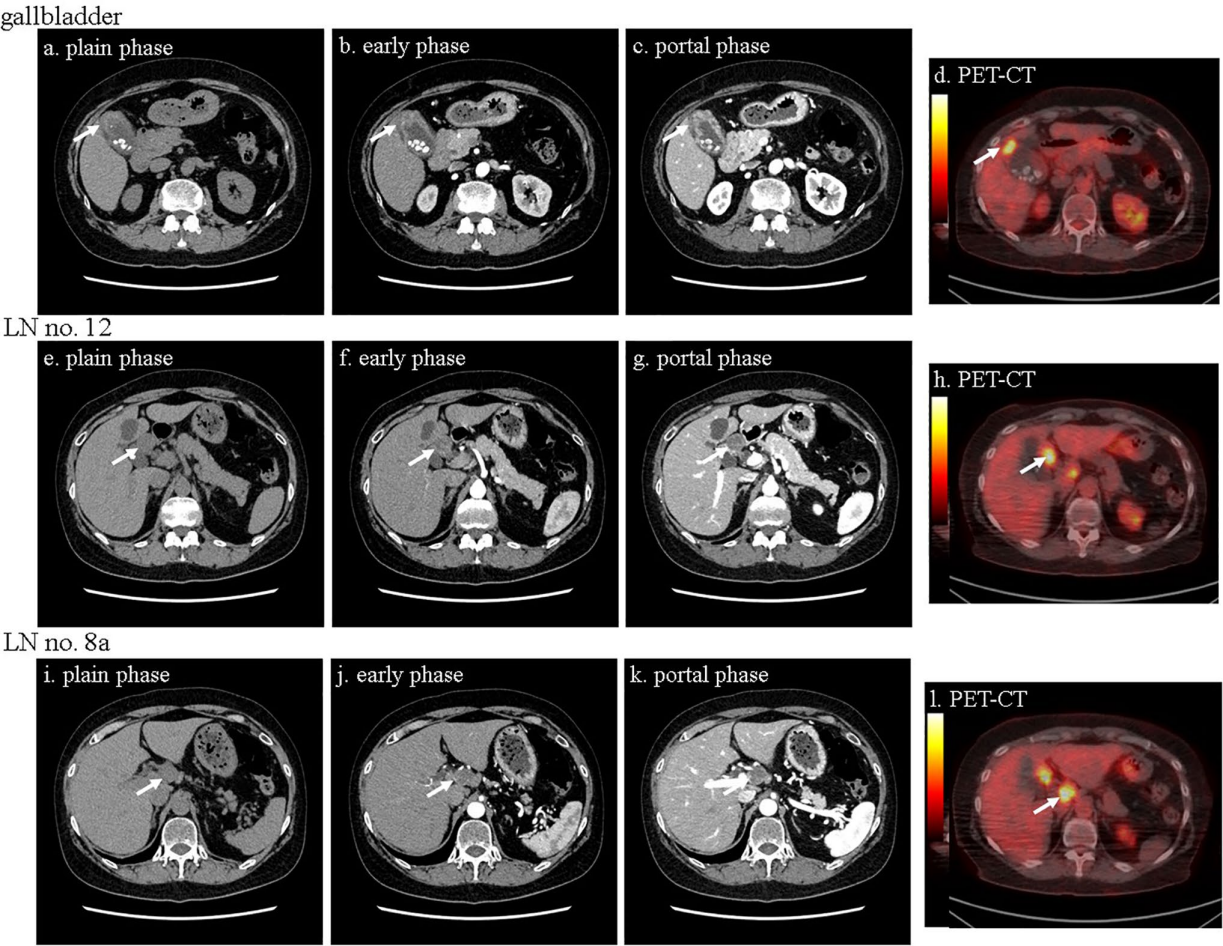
Dynamic CT scan and PET-CT scan findings of the gallbladder. The figure shows dynamic CT scan and PET-CT scan results, 4 months later, taken at the same time as Fig. 1c, f, and i, preoperatively. The irregularly thickened wall showed an increased density in the fundus of the gallbladder on CT scan (a–c). In the LNs No. 12 and 8a, the cortex of the LNs were slightly enhanced, with non-homogenous contrast enhancement seen within both LNs (e–g, i–k). PET-CT scan showed increased ^18^F-FDG uptake in the fundus of the gallbladder, LNs no. 12, and no. 8a (d, h, l)
